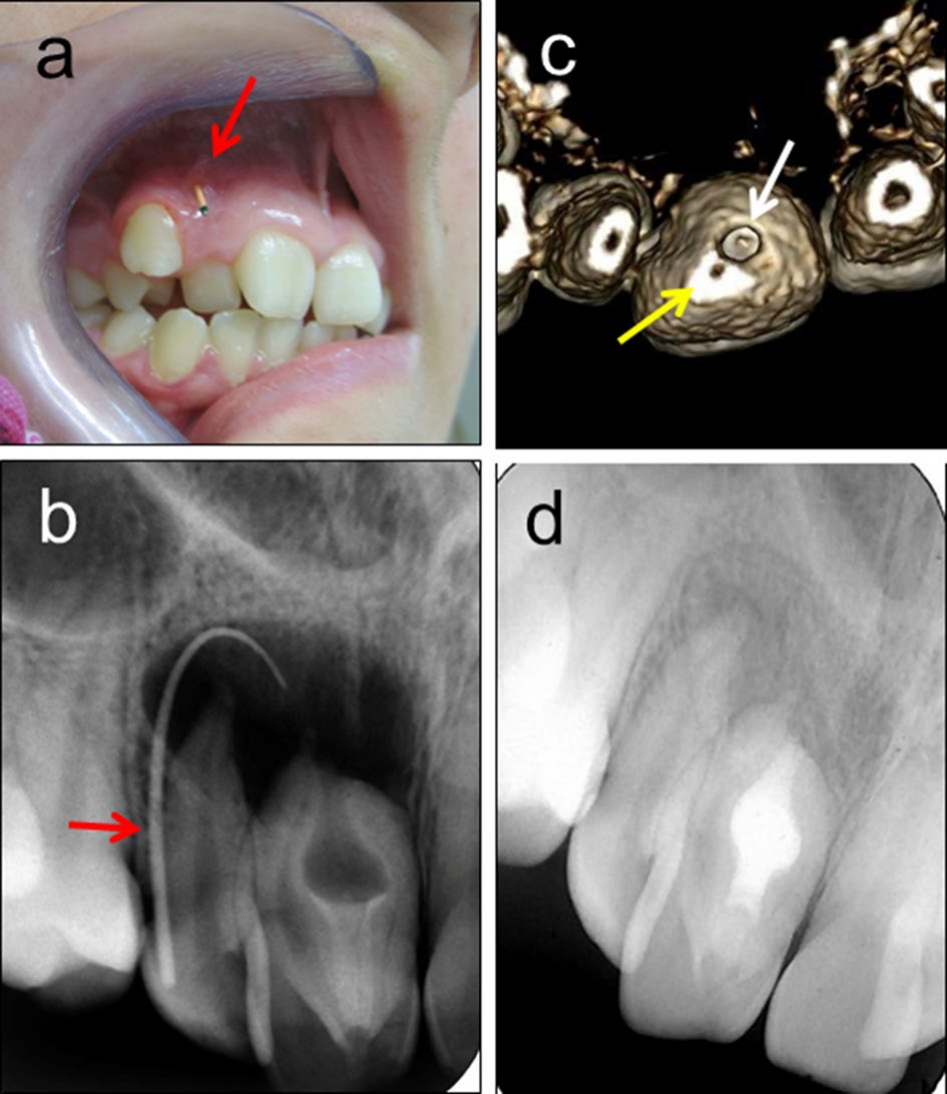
A rare case of Type III dens invaginatus with complications. This rare case of dens invaginatus was not diagnosed until the patient presented with a large periapical lesion with sinus tract [(a,b) Red arrows: a gutta percha point inserted into a sinus tract]. Both teeth #11 and #12 responded positively to cold stimuli, indicating vital pulps. The lesion resulted from bacterial contamination of the lumen of the Type III dens invaginatus which had an independent apical opening [(c) 3D reconstruction from CBCT]. Yellow arrow: the apical opening of the root canal. White arrow: apical opening of the dens invaginatus. Disinfection and obturation of the lumen of the dens invaginatus allowed for healing of the periapical lesion (d). Both teeth remained vital. (Adapted from: Kfir et al. *International Endodontic Journal*, 46:275–288, 2013).
